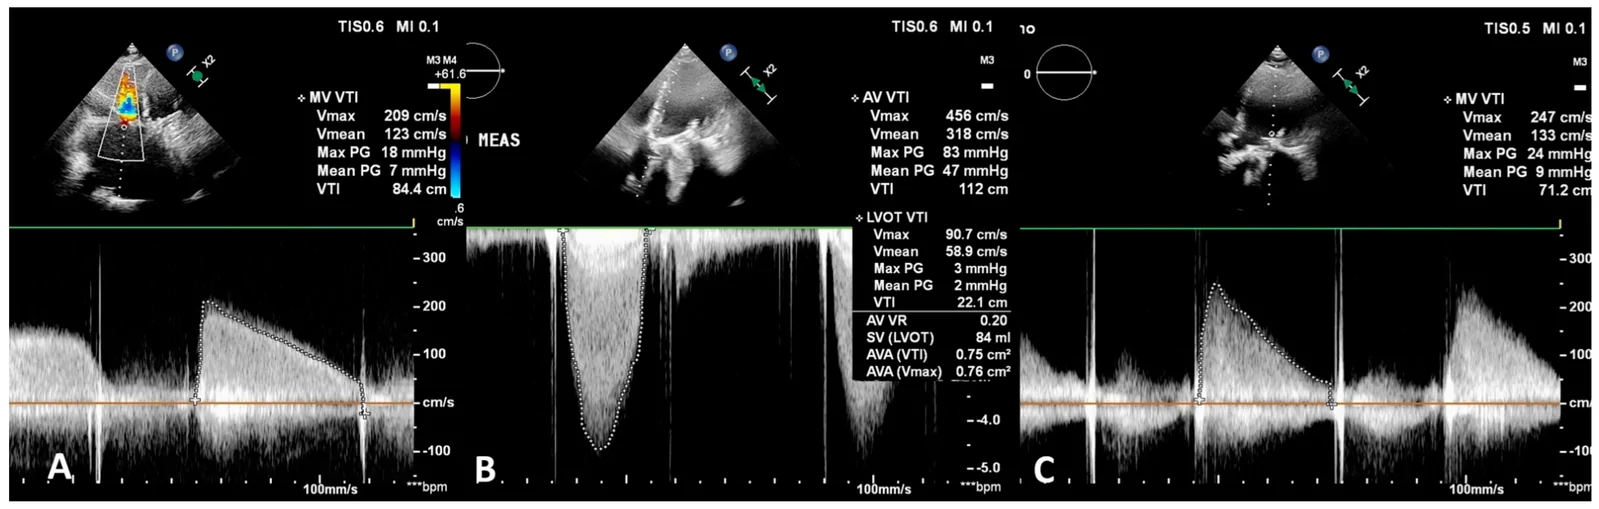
Preprocedural transthoracic echocardiography (apical views). (A) Continuous-wave Doppler across the tricuspid bioprosthesis, showing a mean gradient of 7 mmHg (peak velocity 2.1 m/s), consistent with severe prosthetic stenosis. (B) Continuous-wave Doppler across the native aortic valve, showing a peak velocity of 4.56 m/s and a mean gradient of 47 mmHg; pulsed-wave Doppler of the left ventricular outflow tract (LVOT) is shown alongside for continuity-equation calculation of an aortic valve area of 0.75–0.76 cm^2^, consistent with critical aortic stenosis. (C) Continuous-wave Doppler across the mechanical mitral prosthesis, showing a mean gradient of 9 mmHg, consistent with moderate prosthetic stenosis. * bpm = beats per minute.
